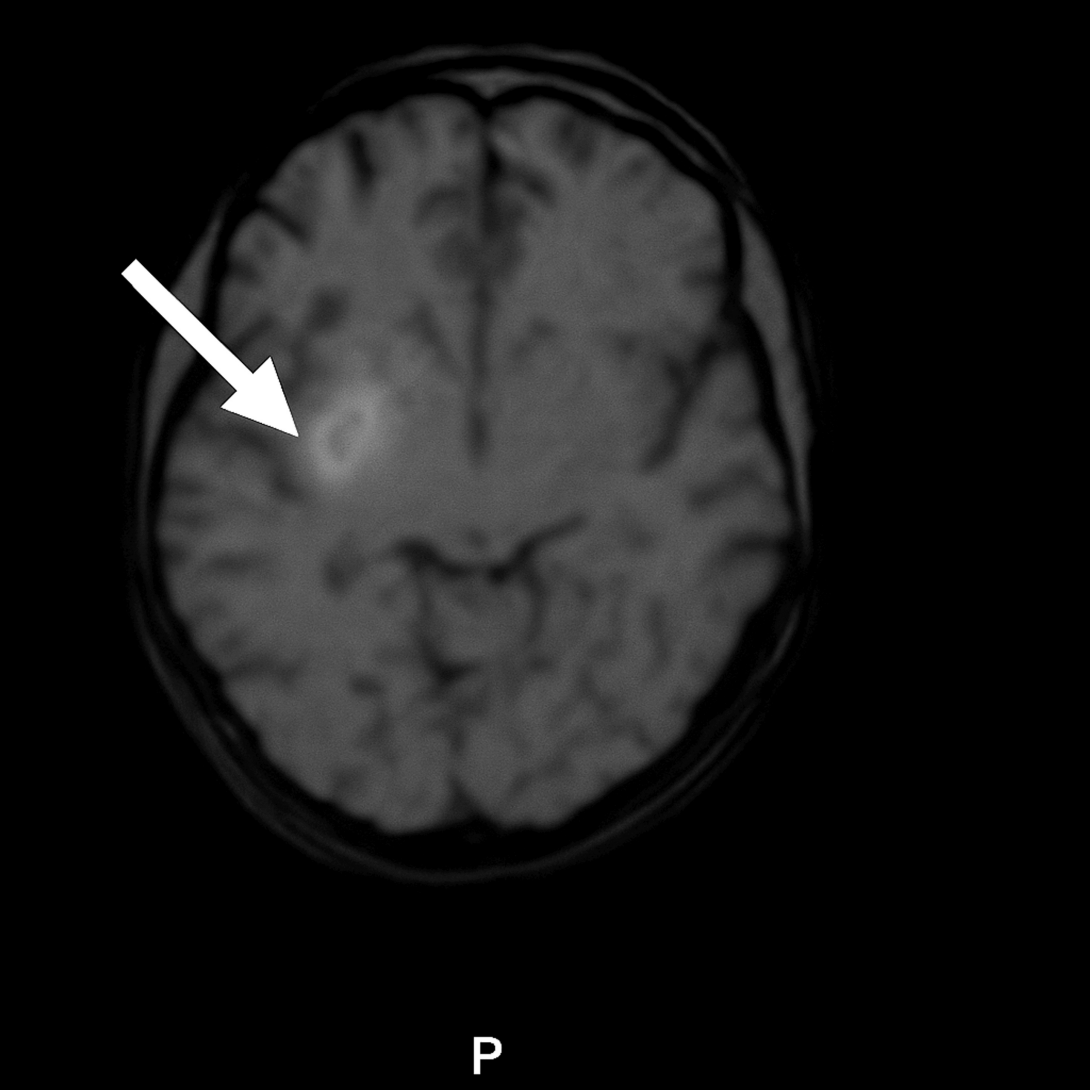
Follow-up MRI showing persistent right basal ganglia haematoma
